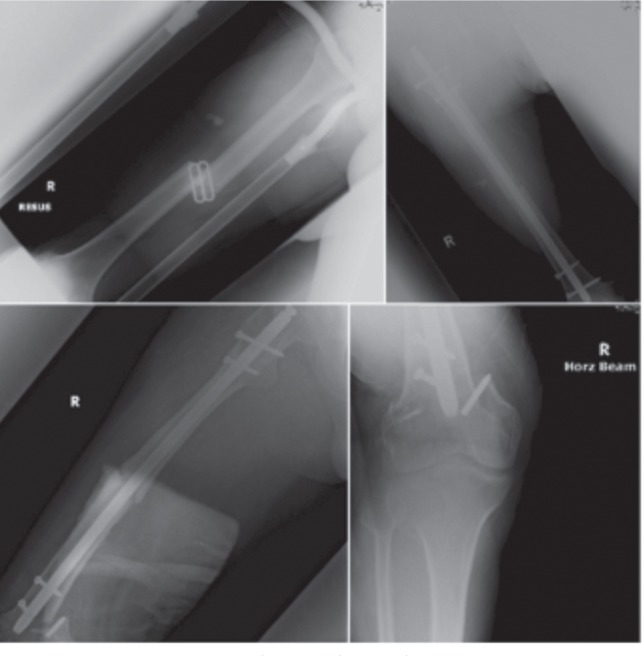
(i) Radiograph of initial femur fracture (ii) Radiograph post intramedullary nail insertion
